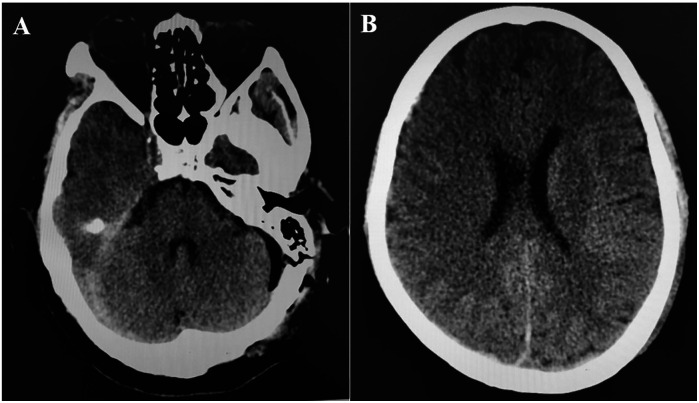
(A,B) CT at 21 h post-operation showed that the right subdural hematoma was significantly dissipated.
